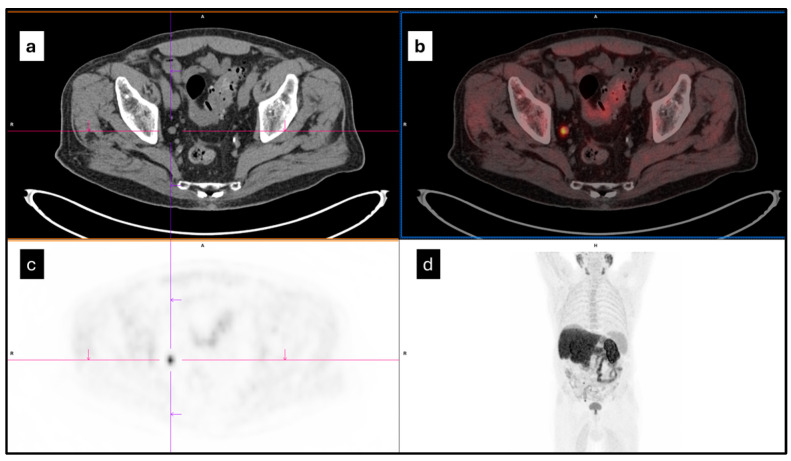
Axial images of CT (a), PET/CT (b), PET (c), and MIP (maximum intensity projection) (d) of a 81-year-old man created by ^18^F-choline PET/CT. A focal ^18^F-choline uptake (SUVmax = 15.6) was identified in the right obturator node.
